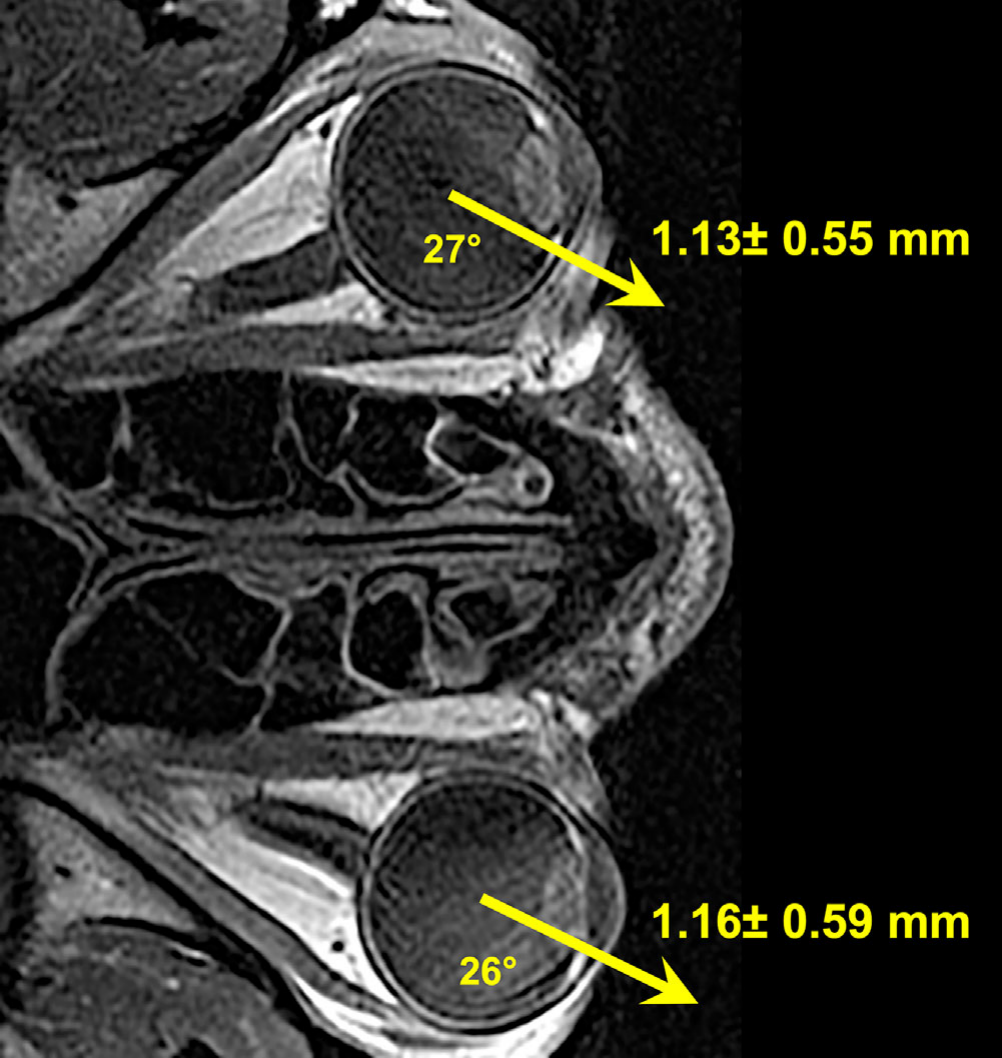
Summary of mean ± standard deviation of globe translation from a reference supine position to a lateral decubitus position, as superimposed on a representative axial T2-weighted MRI image. *Arrows* depict average angles from midsagittal plane. Data are from 20 orbits.
